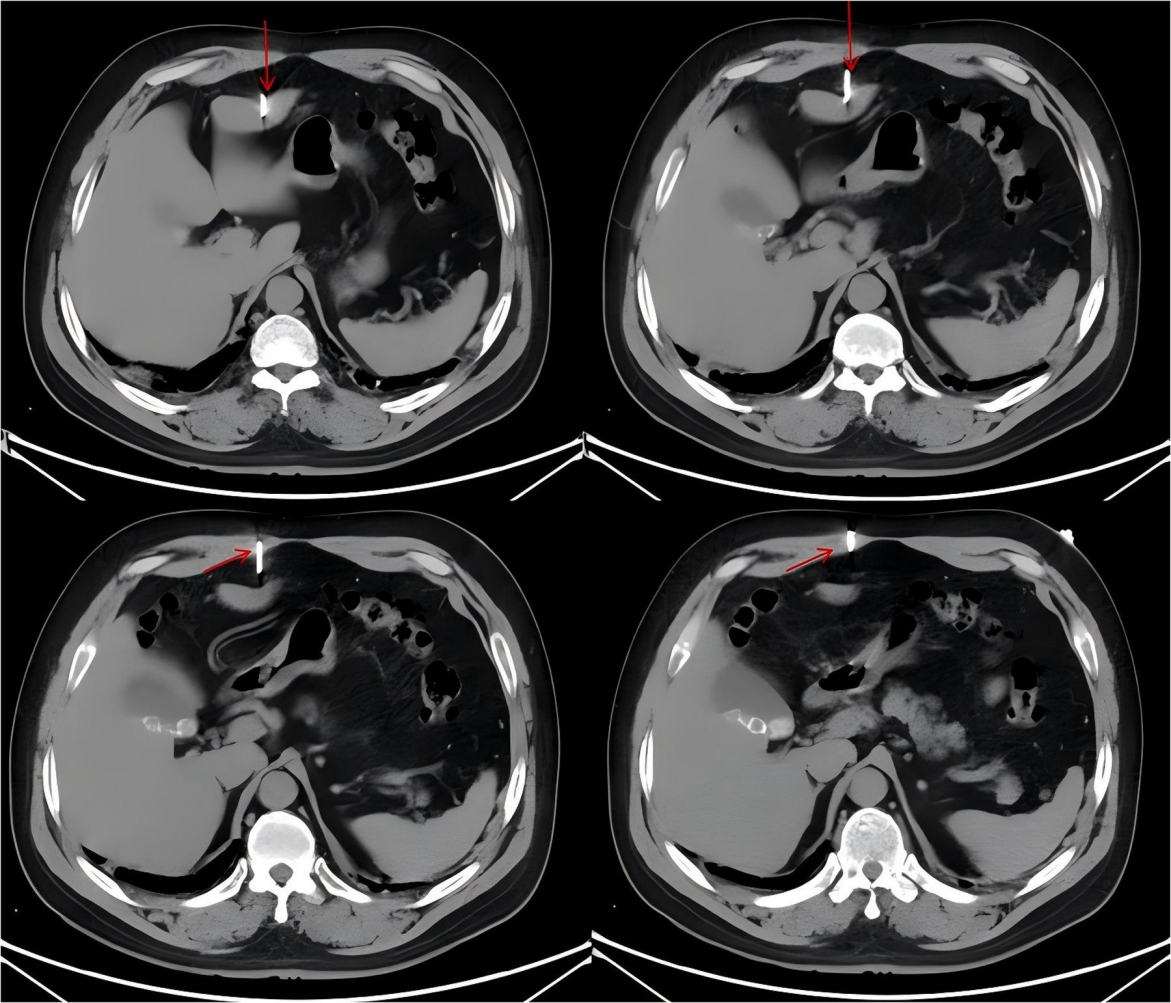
Abdominal CT scan demonstrating penetrating nail injury, red arrow shows the gun nail.
